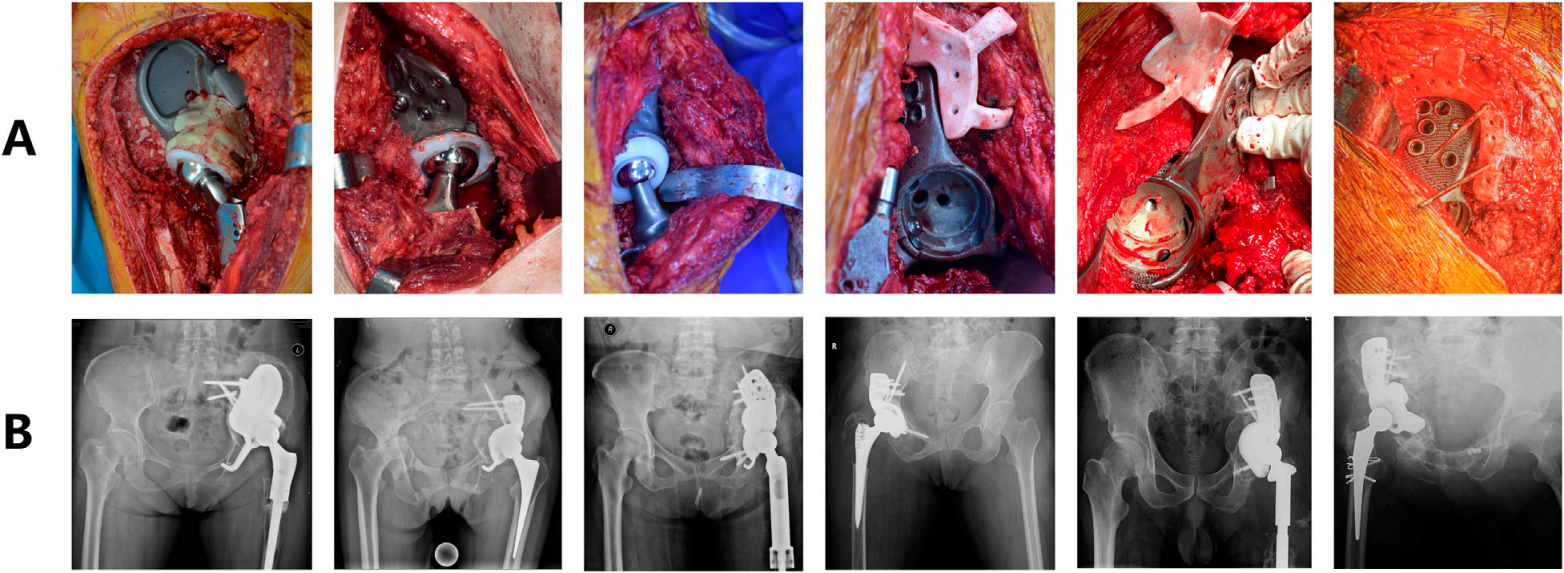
Representative intraoperative surgical field and corresponding postoperative plain radiographs of selected patients. (A) Intraoperative photograph showing prosthesis implantation. (B) Postoperative anteroposterior pelvic radiograph of the patient.
